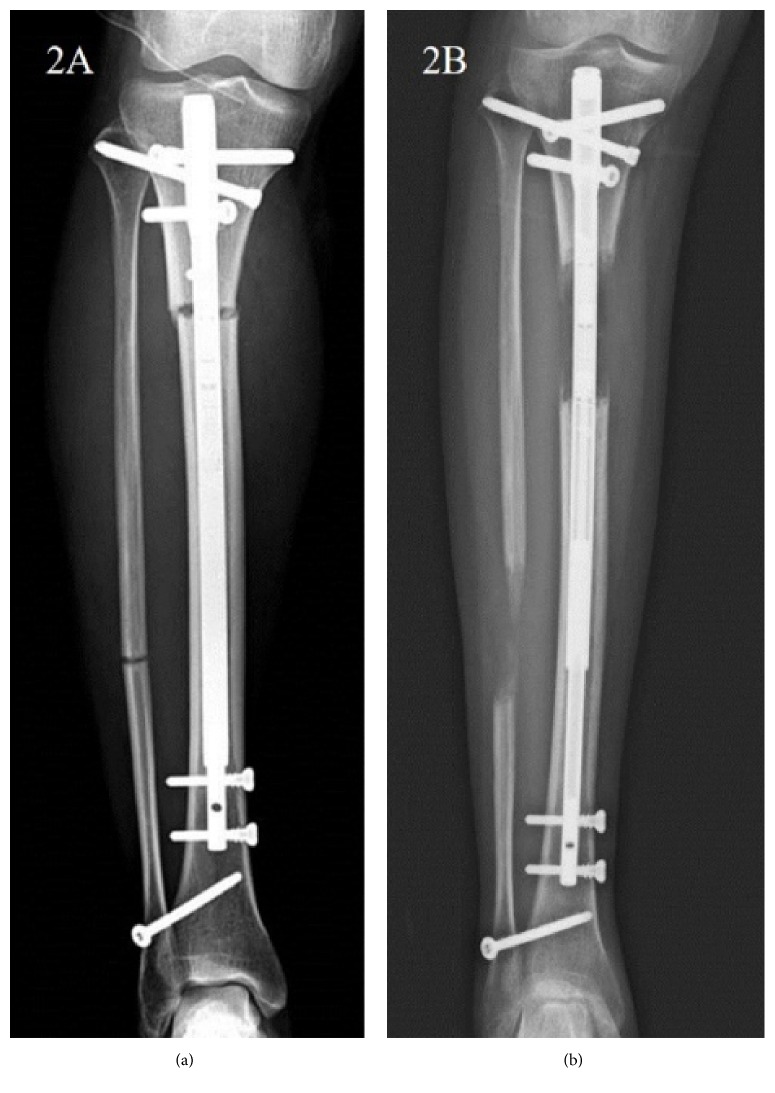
(a) The immediate postoperative plain radiograph showed straight tibia PRECICE nail. (b) The tibia PRECICE nail was bent without breakage after 2 months of lengthening. This was categorized as stability related problem (IIa).
